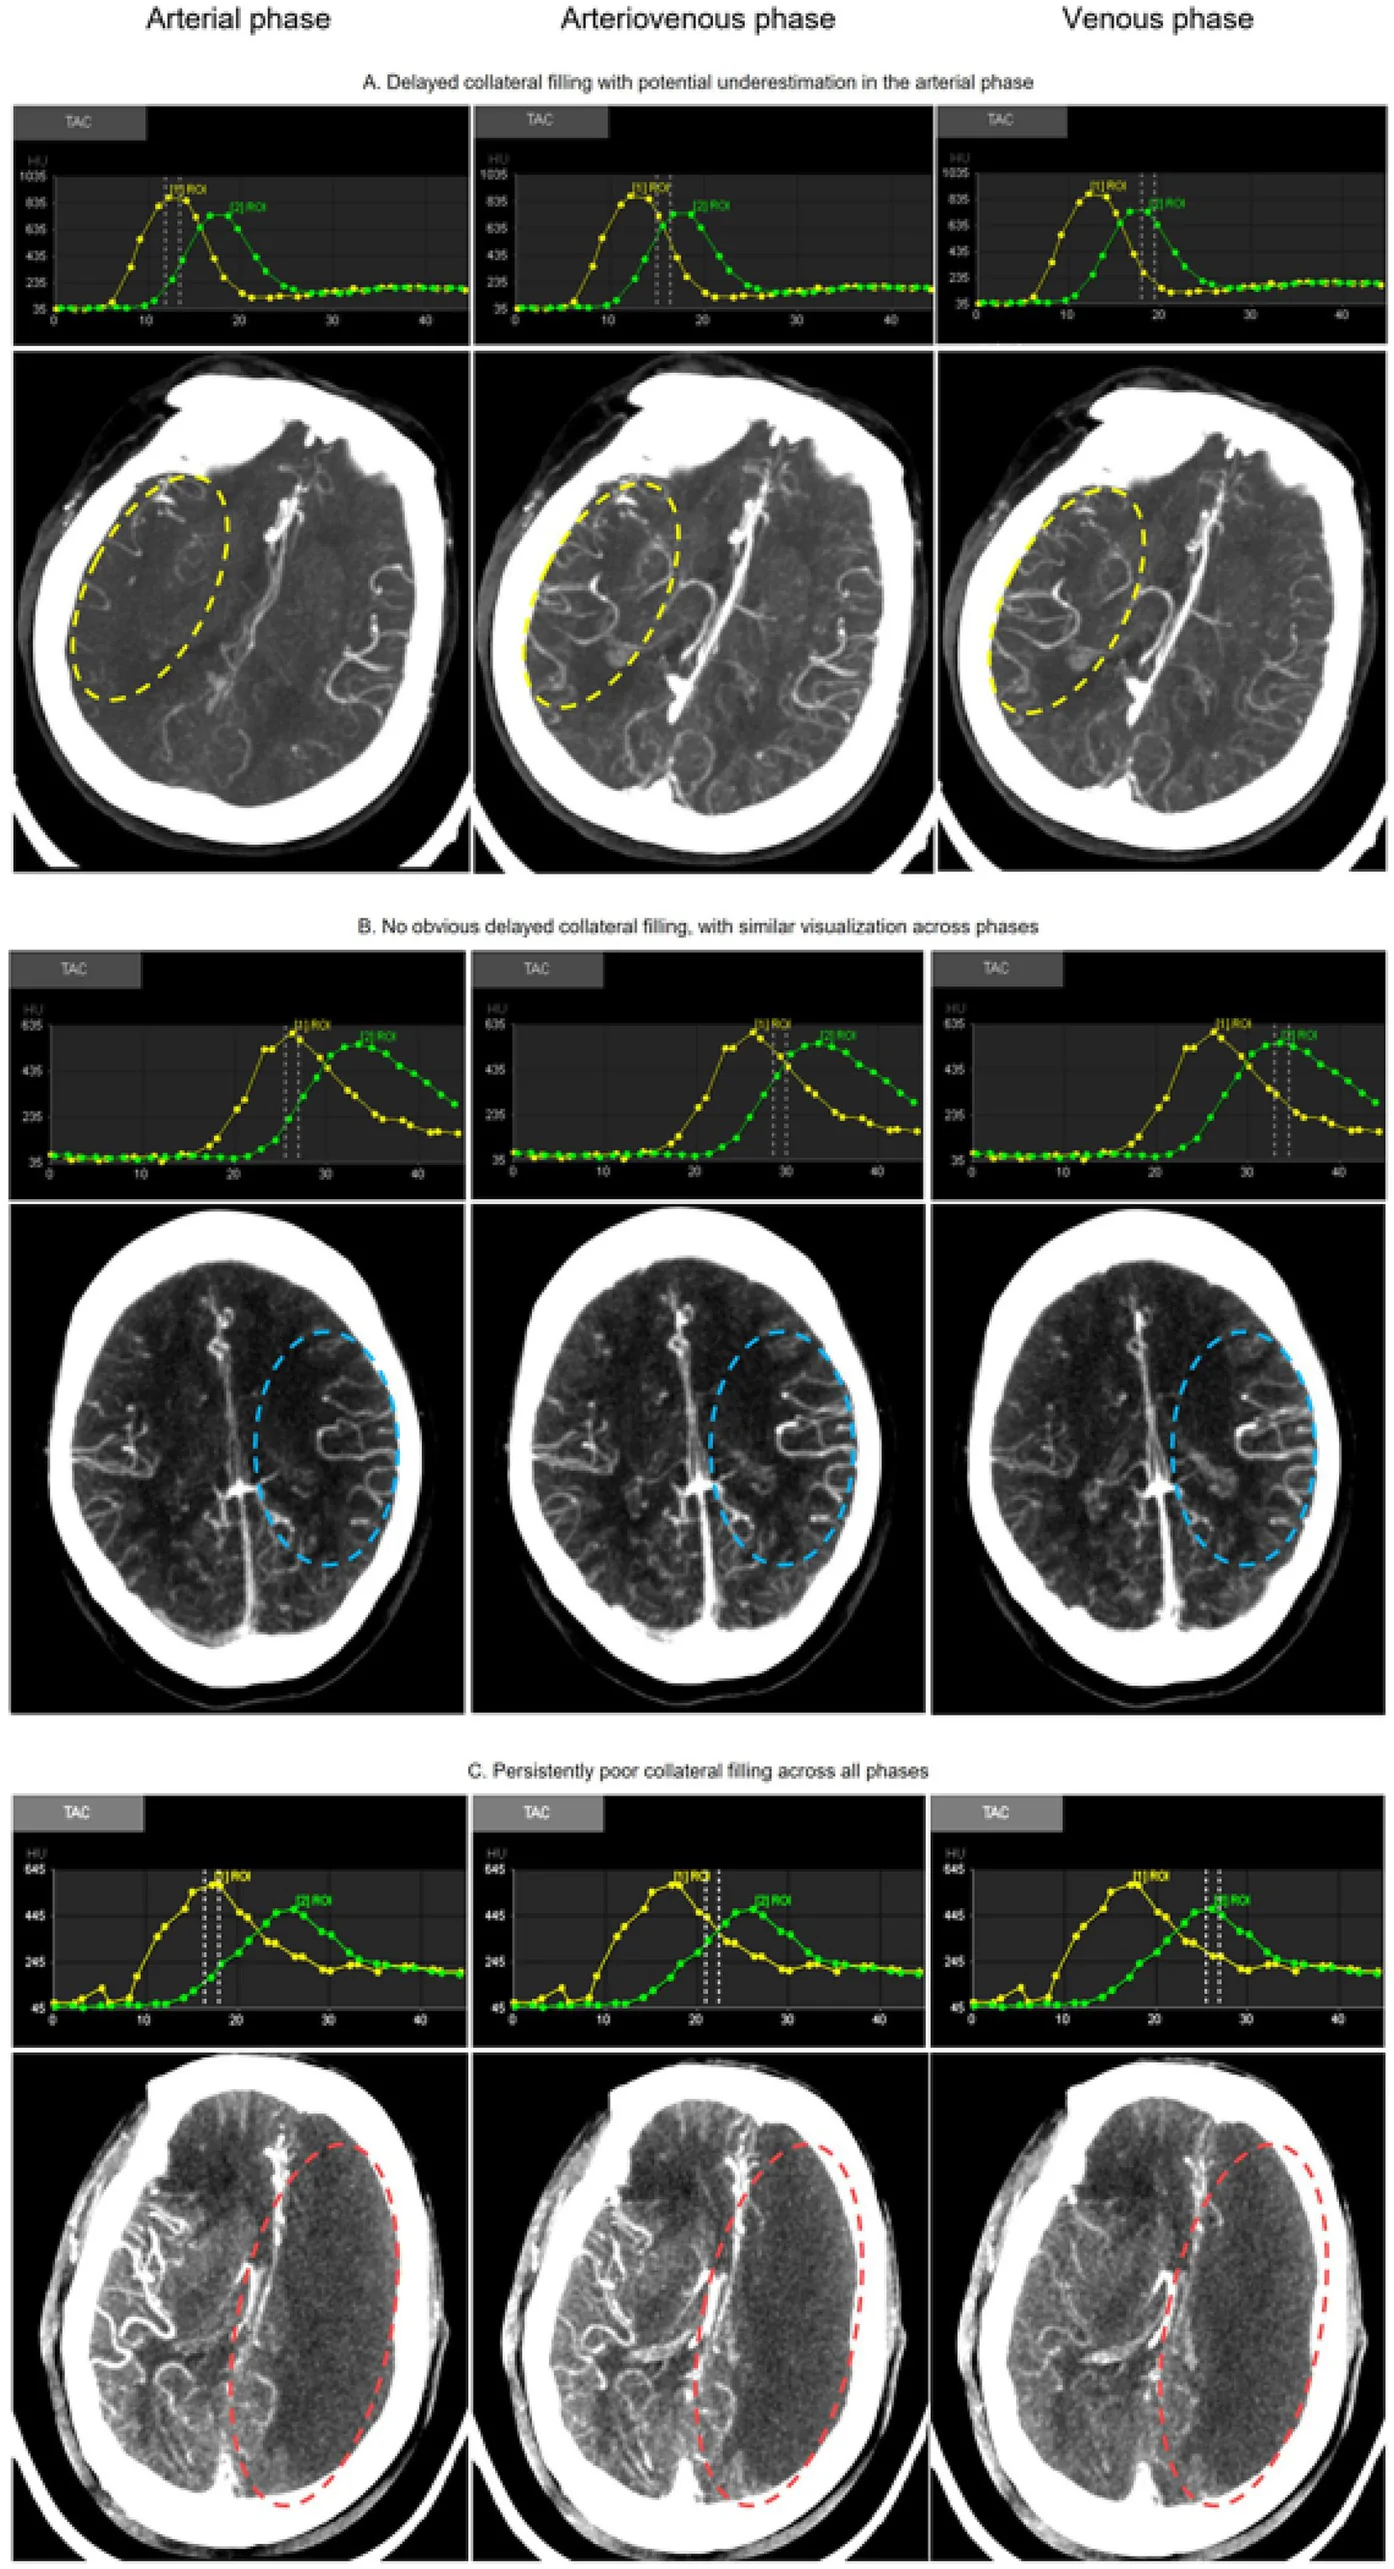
Representative patterns of time-specific Tan-based collateral assessment on CTP-derived CTA. The yellow curve represents the arterial ROI time-attenuation curve, and the green curve represents the venous ROI time-attenuation curve. CTA images were reconstructed at the arterial, arteriovenous, and venous phases according to the selected TAC time points. Row (A) shows delayed collateral filling, in which collateral vessels are poorly visualized in the arterial phase but become more evident in later phases, suggesting potential underestimation by early arterial-phase or single-phase assessment. Row (B) shows no obvious delayed collateral filling, with similar collateral visualization across phases. Row (C) shows persistently poor collateral filling across all phases, indicating that venous-phase assessment does not artificially upgrade the collateral score when effective collateral filling is absent. CTP, computed tomography perfusion; CTA, computed tomography angiography; TAC, time-attenuation curve; ROI, region of interest.
